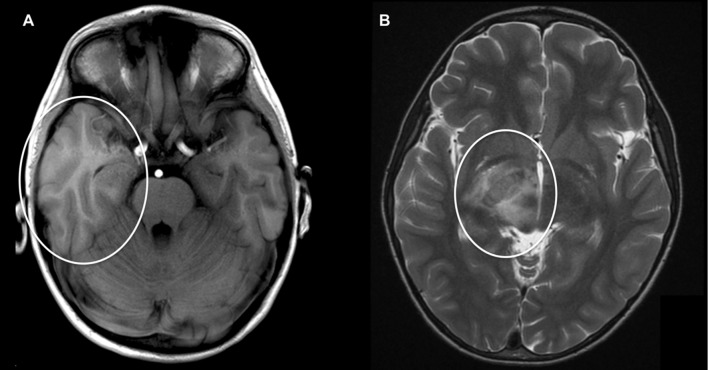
M. pneumoniae*-associated encephalitis. Axial cranial magnetic resonance imaging (MRI) in two children with encephalitis during *M. pneumoniae* infection: (A) 5-year-old boy with hyperintensity and generalized edema of the right temporal lobe [T1 weight MRI; patient 1 published in Meyer Sauteur et al. (2016)]. (B) 9-year-old boy with generalized edema of crus posterior of capsula interna [T2 weight MRI; reprinted with permission from Meyer Sauteur et al. (2014c)].
